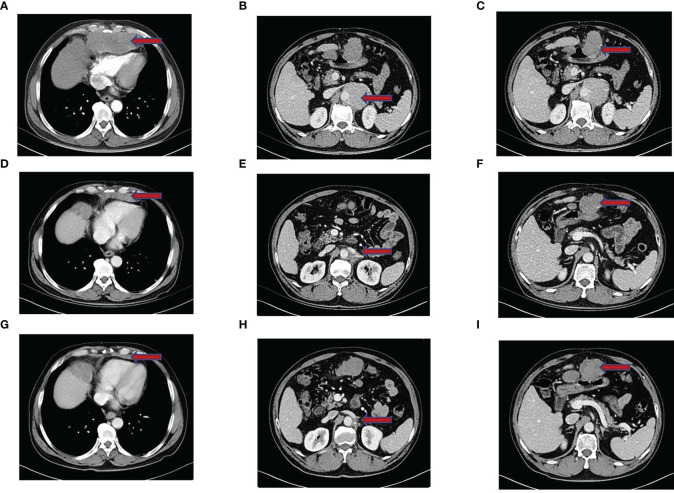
Imaging performance. (A–C) Enhanced CT of the chest and abdomen before treatment showed a dense mass shadow of soft tissue in the anterior mediastinum, multiple retroperitoneal lymph nodes enlarged and fused into a mass, and left liver occupancy. (D–F) After 2 months of tislelizumab combined with epirubicin treatment, the CT showed a significantly smaller lesion shadow than before, and the efficacy evaluation was PR. (G–I) In April 2022, Chest CT showed that the size of the anterior mediastinal hypodense foci was similar to that before. CT of abdominopelvic cavity showed multiple posterior peritoneal and perihepatic occupations with increased lesion extent compared to previous CT, and some of the lesions were new. Suspicious new nodules in segment VI of the liver, and efficacy evaluation of PD.
